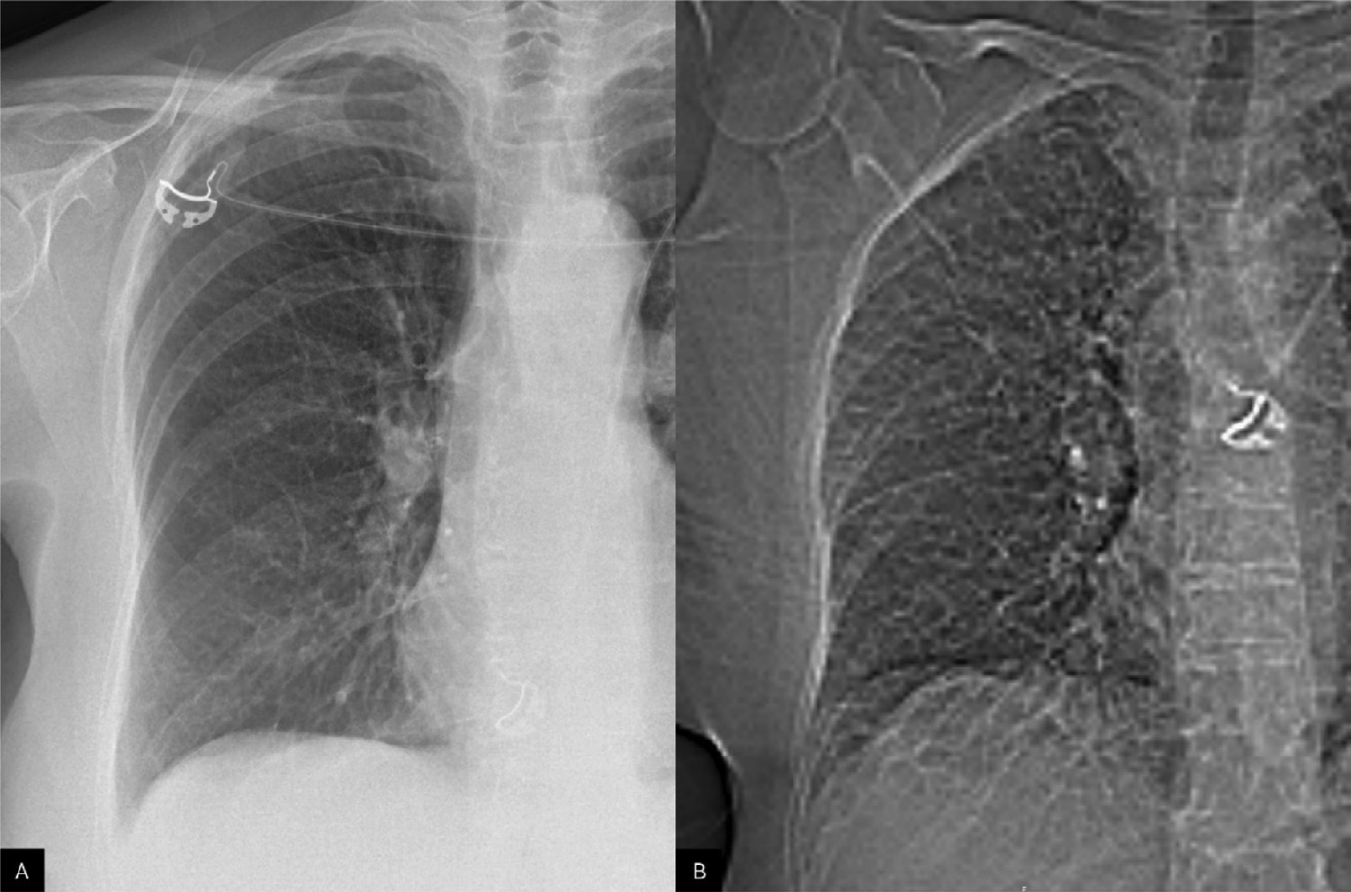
Comparison between the admission chest X‑ray (A) and the scout view from the CT scan performed two hours later (B), showing the onset of linear parenchymal opacities in the peripheral region of the right lung field.
